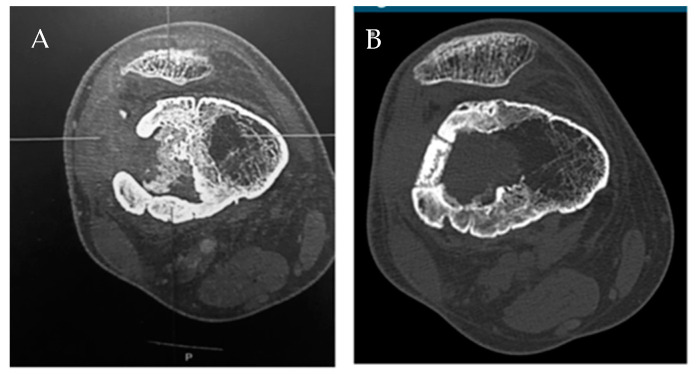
Image (A): pre-operative axial CT scan of the femur. Bone loss and bone remodeling as a result of chronic infection (osteomyelitis) is seen. Image (B): axial CT scan after follow-up of 11 months. Tight contact between bone and porous alumina ceramic is seen and demonstrates appropriate biocompatibility and osseointegration. Reprinted from Fiorenza et al. [144] following the Creative Commons Attribution (CC BY) license (http://creativecommons.org/licenses/by/4.0/) (accessed on 16 September 2021).
